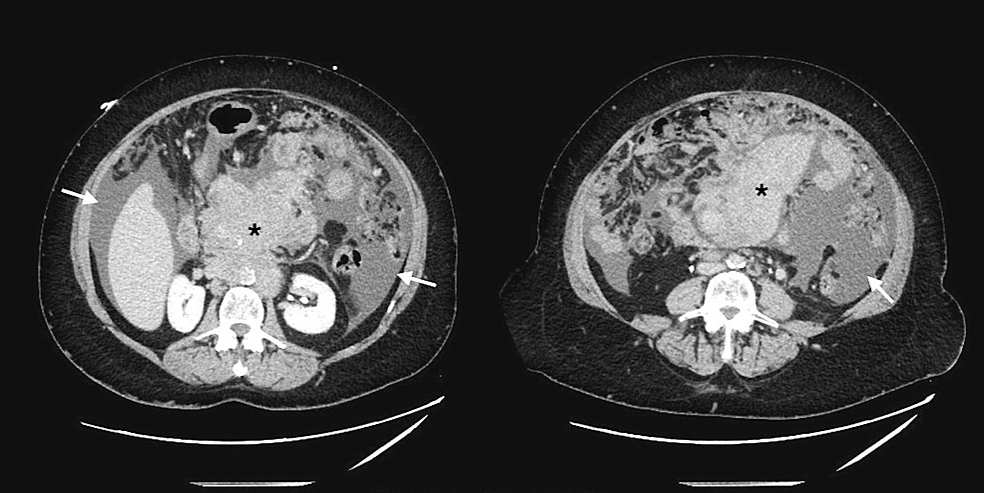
Computed tomography of the abdomen (axial section) CT abdomen demonstrates a large midabdominal mass (black asterisks) and large ascites (white arrows).
